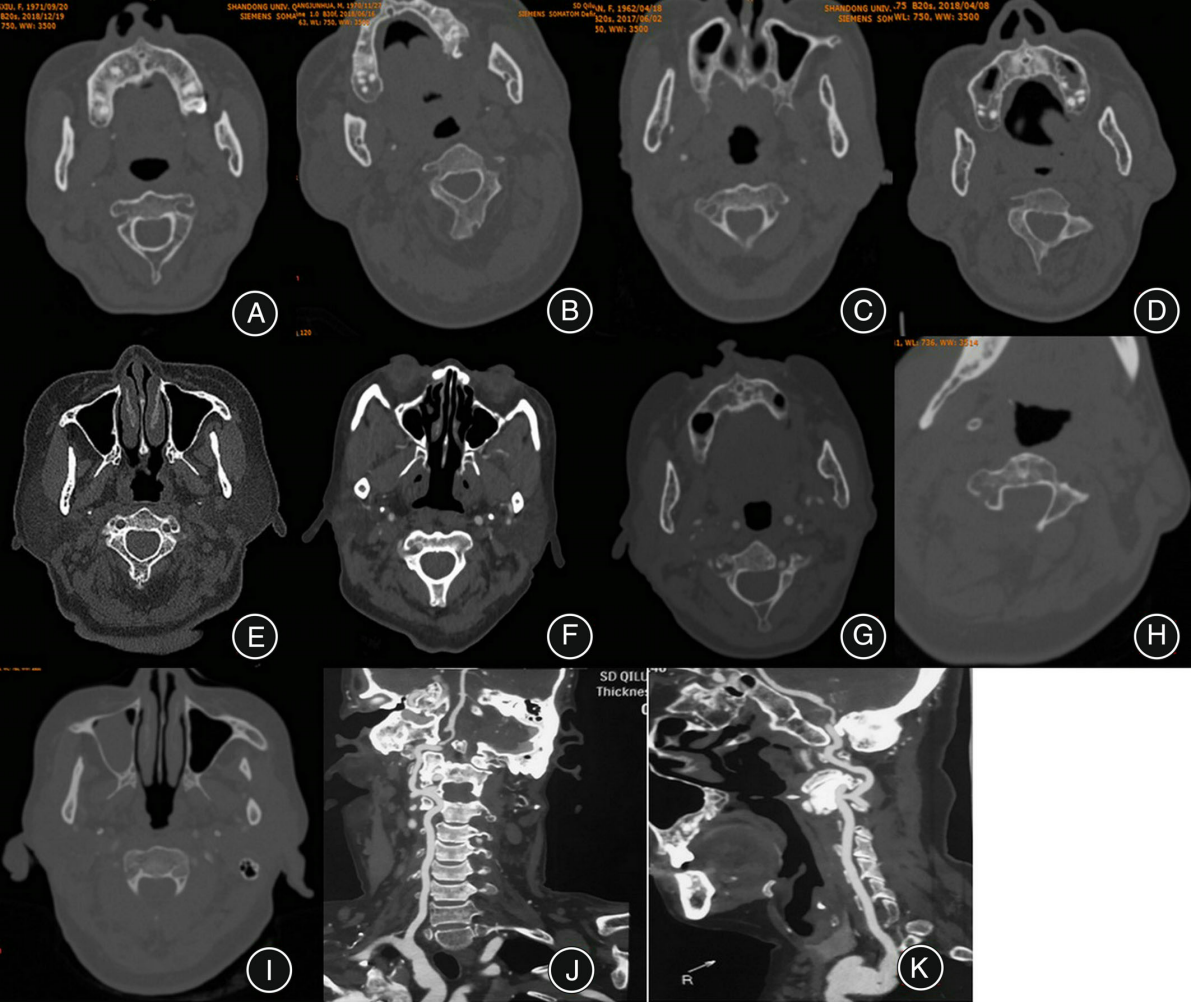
The CT scan of cervical spine in basilar invagination patients. (A) Type I, the axis lateral mass and pedicle are normal. (B) Type IIA, the axis right lateral mass is dysplasia. (C) Type IIB, the axis bilateral lateral masses are all dysplasia. (D) Type IIIA, the axis left pedicle is dysplasia. (E) Type IIIB, the axis bilateral pedicles are all dysplasia. (F) Type IVA, the axis left lateral mass and right pedicle are all dysplasia. (G) Type IVB, the axis bilateral lateral masses and left pedicle are all dysplasia. (H) Type IVC, the axis bilateral pedicles and right lateral mass are all dysplasia. (I) Type IVD, the bilateral axis pedicle and bilateral lateral masses are all dysplasia. (J, K) The coronal and sagittal plane of the high span of the axis vertebral artery.
